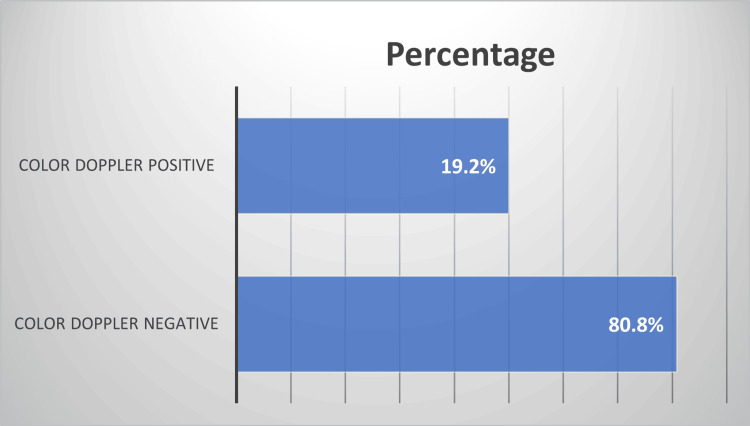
The percentage (%) of colour Doppler in patients with CTS CTS: carpal tunnel syndrome
